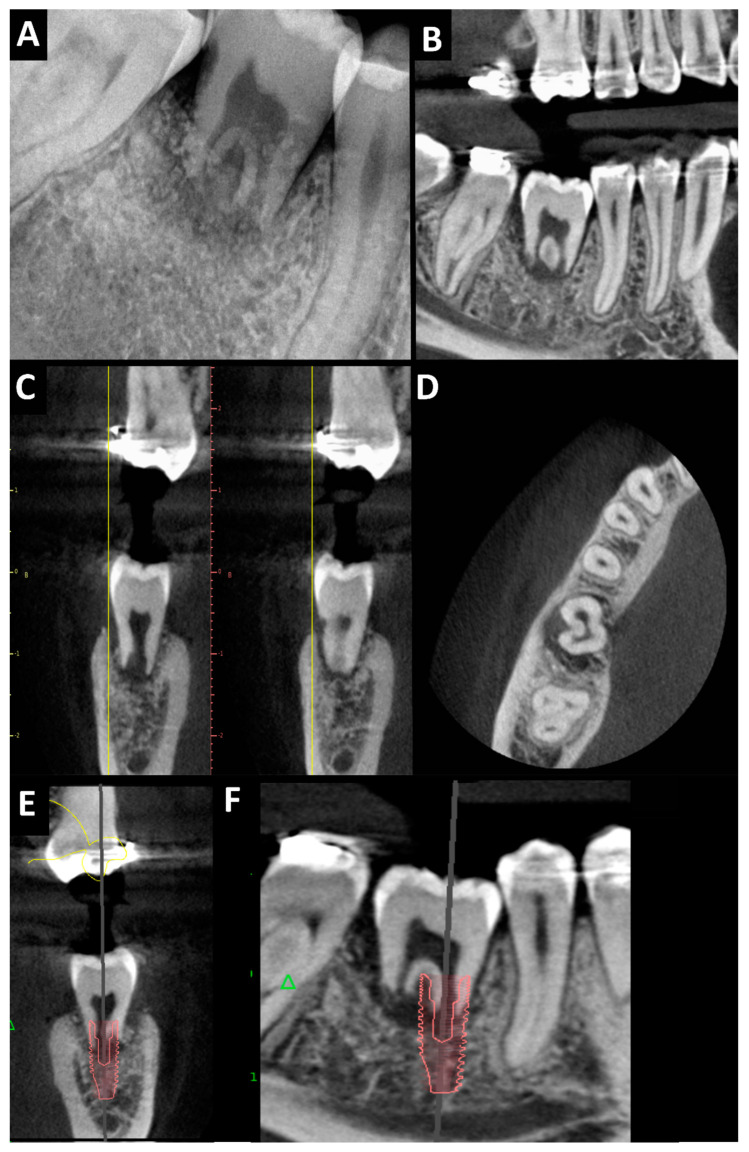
Pulp necrosis and reconstruction of the bone defect—diagnostic imaging one year after the surgical procedure showing cervical inflammatory resorption, unchanged pulp canal dimensions, absent root growth, periapical radiolucency, lack of apical and marginal healing, obtaining interproximal bone with its normal level, and bone presence at the labial and lingual side of the transplanted molar: (A) dental X-ray—tooth no. 38 in place of tooth no. 46; CBCT after 1 year—tooth no. 38 in place of tooth no. 46; (B–D): (B) pantomography reconstruction; (C) cross-sectional view; (D) axial view—visible bone healing; implant planning (E,F): (E) cross-sectional view; (F) orthopantomography reconstruction (planed implant: ø 4.2 mm, H 13 mm).
